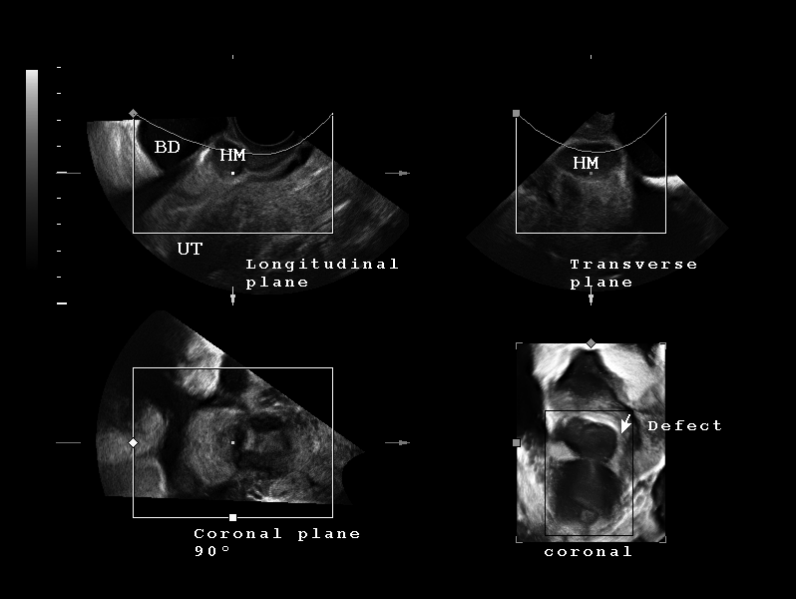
Three-dimensional transvaginal ultrasound scan (in multiplane acquisition mode) of the uterus-hematoma-bladder complex (UT, HM, BD respectively). Please note that the white pixel (placed in the center of each image) always correspond with the same space point of the three orthogonal planes, and is located referring HM, between UT (at the level of the uterine scar) and just beneath BD. Defect's surface three-dimensional reconstruction (of the coronal plane) correspond with bottom right picture, and is framed with a white arrow (instead of white pixel).
